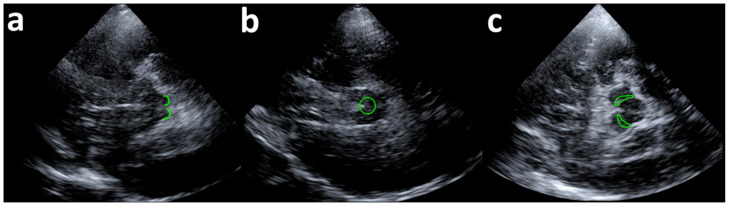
Ultrasound visualization of target brainstem nuclei. (a) Superior colliculus (SC); (b) Nucleus raphe magnus (NRM); (c) Substantia nigra (SN). The size of the targeted area was ~15 mm and brainstem nuclei were centred on the screen.
